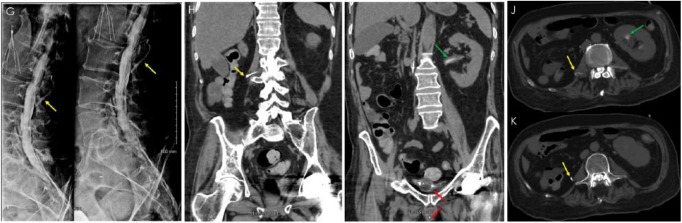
Computed tomography (CT) myelogram. X-ray myelogram with intrathecal iohexol contrast (Omnipaque, GE Healthcare, Buckinghamshire, UK) by fluoroscopy-guided sacral hiatus puncture (G) followed immediately by CT abdomen and pelvis without intravenous contrast (H, I, J, and K). Contrast is visible within an extra-thecal tubular structure beginning at approximately L2-L3 vertebrae (G, H, J, and K—yellow arrows). Contrast is also seen within the bladder surrounding the Foley catheter bulb and within the Foley catheter (I—red arrows), suggesting patent communication between the intrathecal space and the genitourinary system. Contrast in left renal pelvis suggests retrograde flow in the contralateral ureter (I and J—green arrows).
